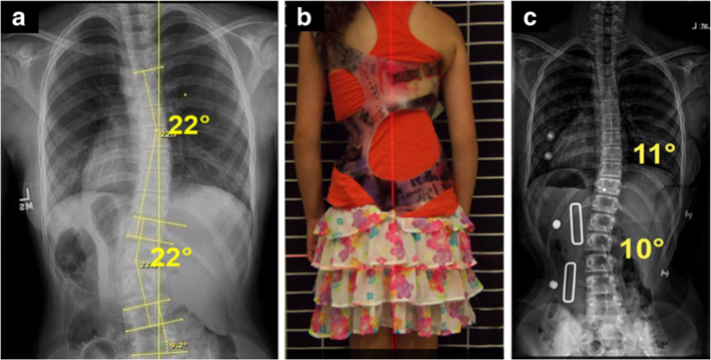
WCR in-brace correction with optimal pad placements. a: Patient out-of-brace and pre- Cheneau-Rigo handmade type brace in July 2013, showing a right thoracic curve of 22-degree Cobb angle at T7 to T12 with the curve apex at T9; and a left lumbar curve of 22-degree Cobb angle at T12 to L4 with the curve apex at L2-3. Although the upper end-plate maximum inclination was T7, the curve inclined up to T7-T6-T5. b: Patient in a Cheneau-Rigo handmade type brace, with correction of decompensation of the trunk and a more balanced pelvis. c: The Cheneau-Rigo handmade type in-brace X-ray presented the thoracic pad at T9-10 with the pad pressures and size of pad covering approximately T8 to T11 and the counterforce at the left axilla at T 5. As a result, the Cheneau-Rigo handmade type in-brace X-ray showed a scoliosis with over 50% correction, which improved from thoracic 22 degrees Cobb and lumbar 22 degrees Cobb out-of-brace to thoracic 11 degrees Cobb and lumbar 10 degrees Cobb in-brace.
